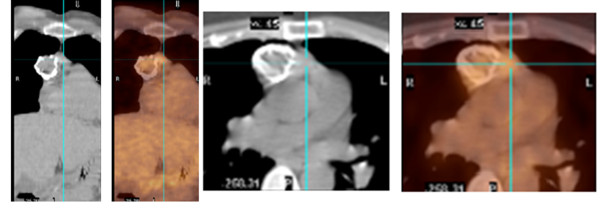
PET/CT scan: 2 views showing the 3.9 cm rim calcified right anterior mediastinal mass which is FDG avid (SUV 6). The adjacent midline anterior mediastinal density is FDG avid as well (SUV 3.9).
